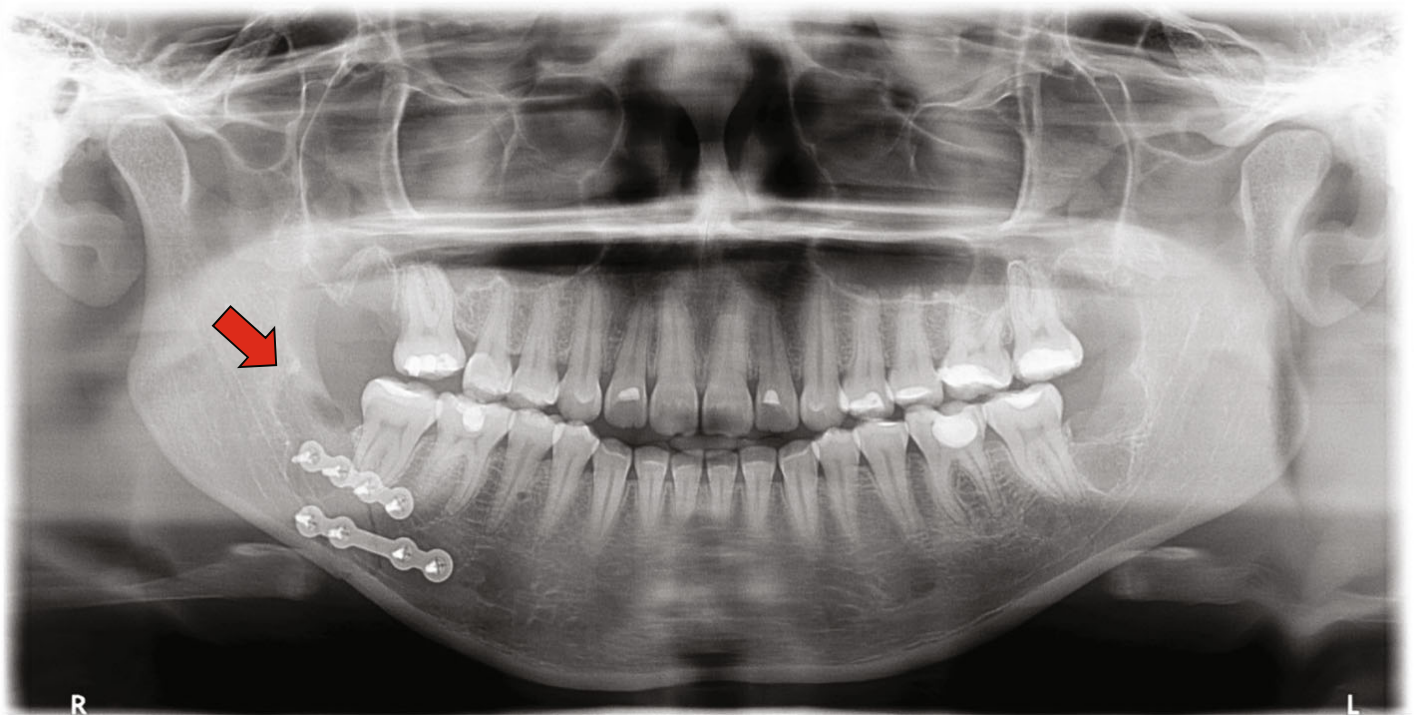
Follow-up panoramic radiograph eight days after the surgery showing reduction and fixation of the fracture (red arrow).
